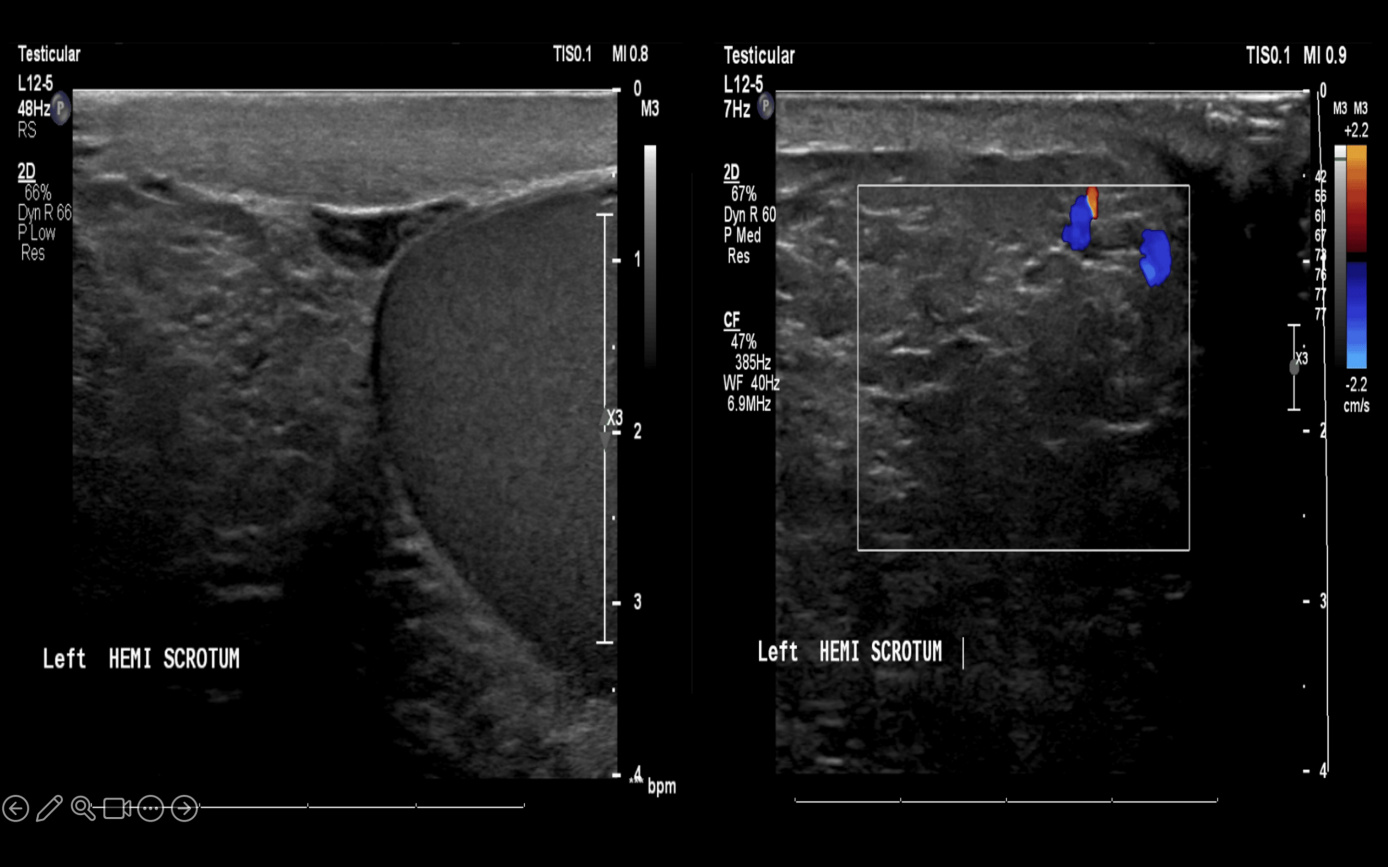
Ultrasound showing left hemiscrotum occupied by echogenicity
